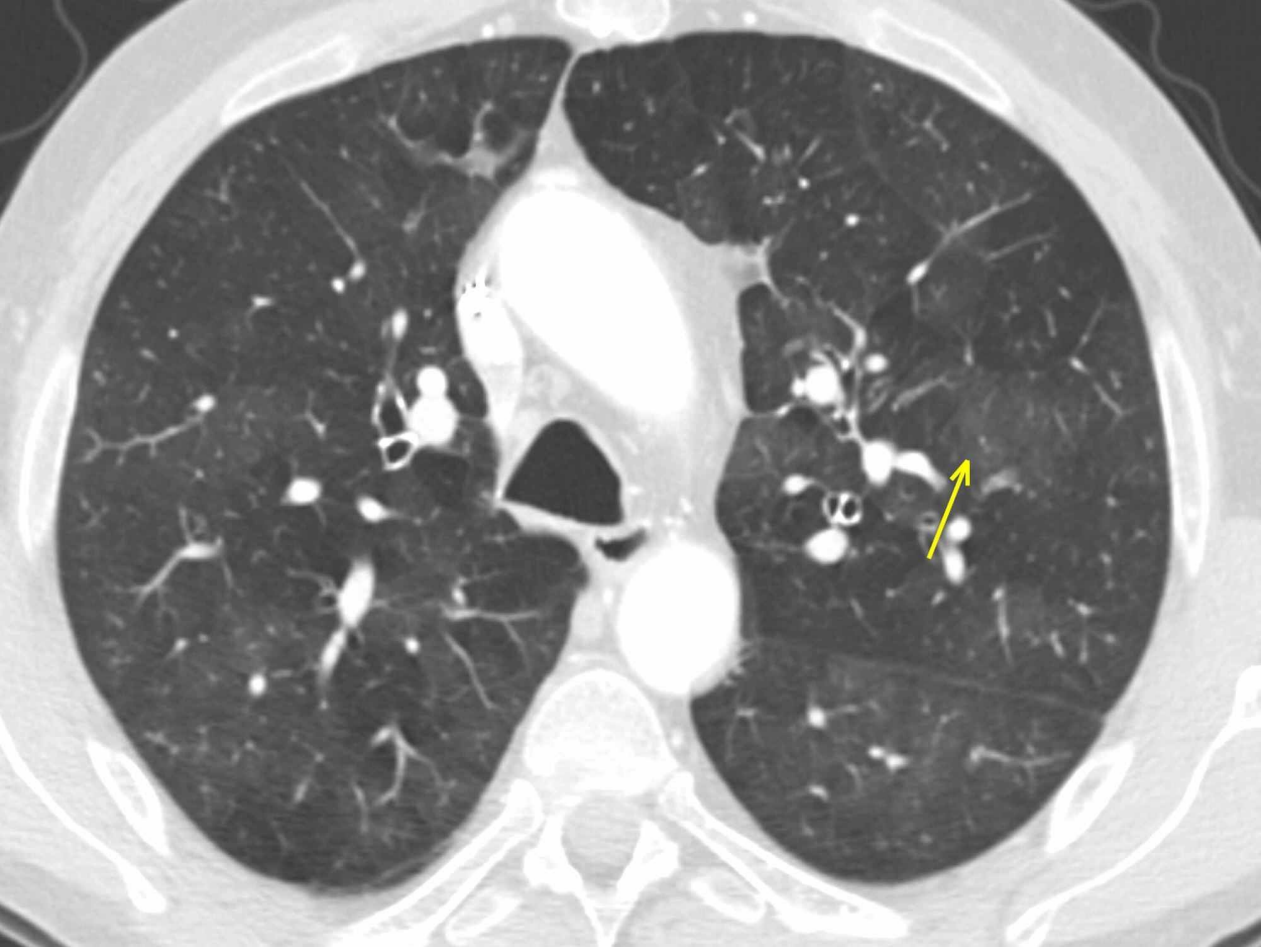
Emphysema The same patient as in Figure 7: a careful review of the CT performed in January 2019 shows identical bilateral GGOs (arrow), confirming imaging features as consistent with emphysema and, therefore, ruling out COVID-19 pneumonia. CT: computed tomography; GGO: ground-glass opacity; COVID-19: coronavirus 2019
